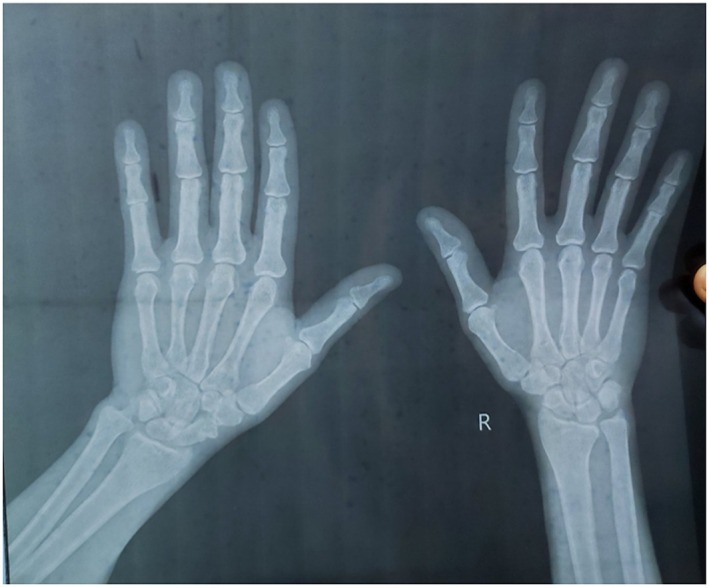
Case 1. Radiographs of the hands and forearms showing no evidence of periosteal reaction, cortical thickening, or other osseous abnormalities.
